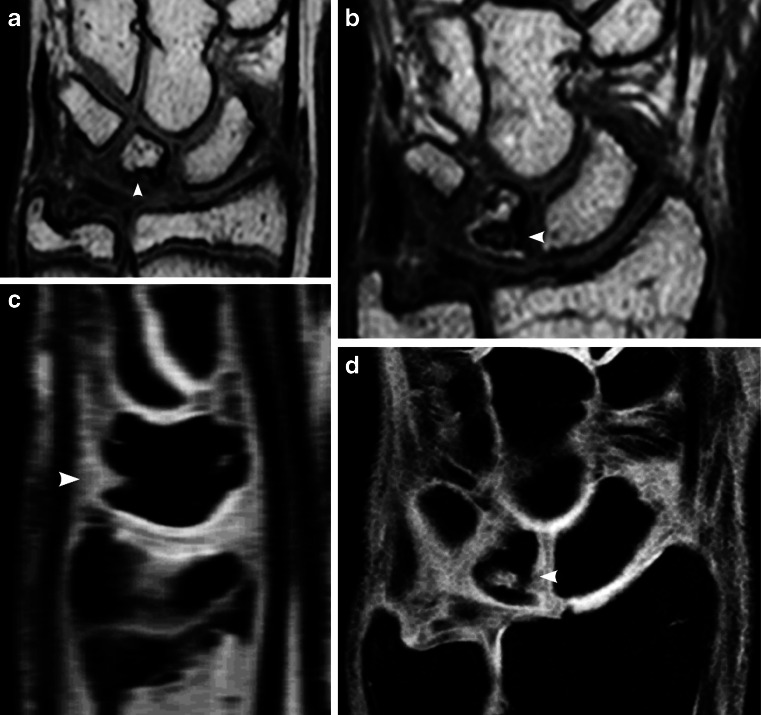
Depressions (*arrowhead*) in the lunate bone. a, b Coronal T1-weighted turbo spin-echo MRI in a 14-year-old boy (a). At 4-year follow-up MRI (b) the depression appears more irregular. c, d Coronal water-selective (coronal volumetric steady-state spoiled gradient echo) cartilage sequence at 4-year follow-up. Sagittal reconstruction (c) and coronal image (d) show intermediate signal on the articular side of the depression, which was therefore classified as not covered. The sagittal reconstruction (c) locates this depression to the dorsal side of the lunate
